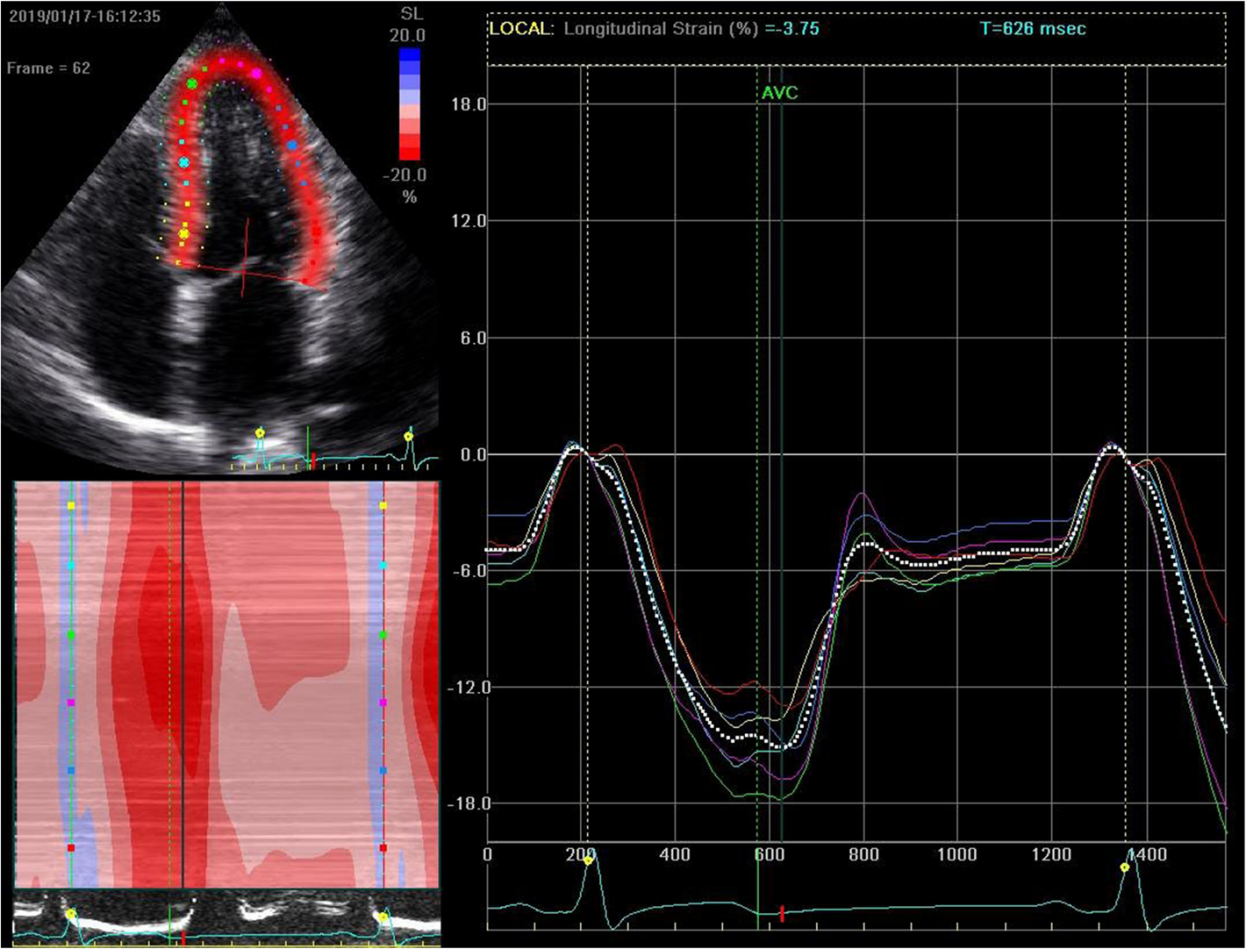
Measurement of GLS of a patient with DN by 2D-STE. The image was obtained from the apical four-chamber view
